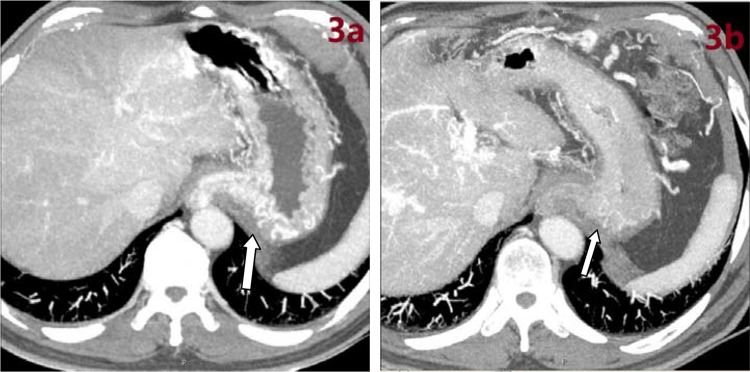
Perioperative enhanced CT images. a) Gastroesophageal varices on preoperative enhanced CT. b) Absence of varicose veins on postoperative enhanced CT after foam sclerotherapy in the same patient.
